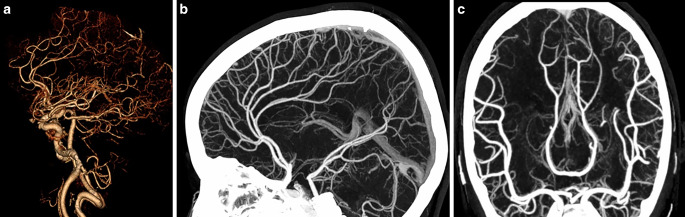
Example of a UHR-CTA of a female 41-year-old patient. a Volume rendering technique in the sagittal plane. b Maximum intensity projection with a slice thickness of 17.5 mm in sagittal view; c Maximum intensity projection with a slice thickness of 17.5 mm in Towne view for tracing the vascular course of the posterior cerebral artery. The superior image quality and the distinct delineation of the peripheral sub-segments are emphasized
